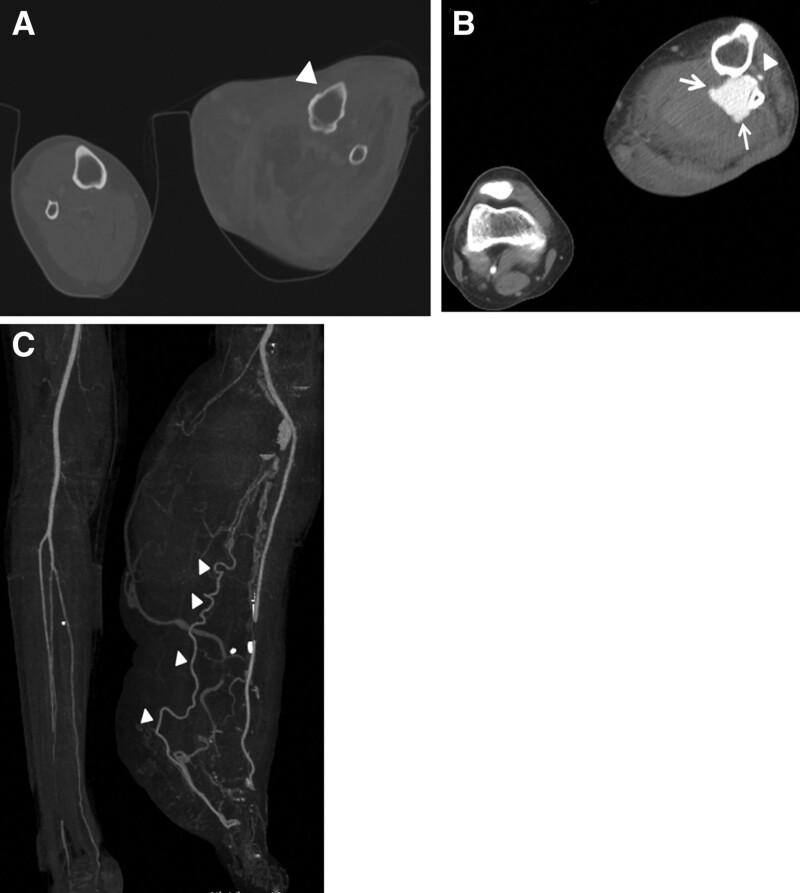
(A) CT bone setting images showing cortical thinning and irregularity (arrowhead) of the left tibia and fibula compared to the right side. (B) Axial CT image showing a pseudoaneurysm (arrow) in the left proximal ATA (arrowhead) and adjacent hematoma. (C) 3D reconstruction CT image in which the PTA and peroneal artery are not visible, and the foot and leg flow is maintained by the collateral flow of the ATA (arrowhead). ATA = anterior tibial artery, CT = computed tomography.
